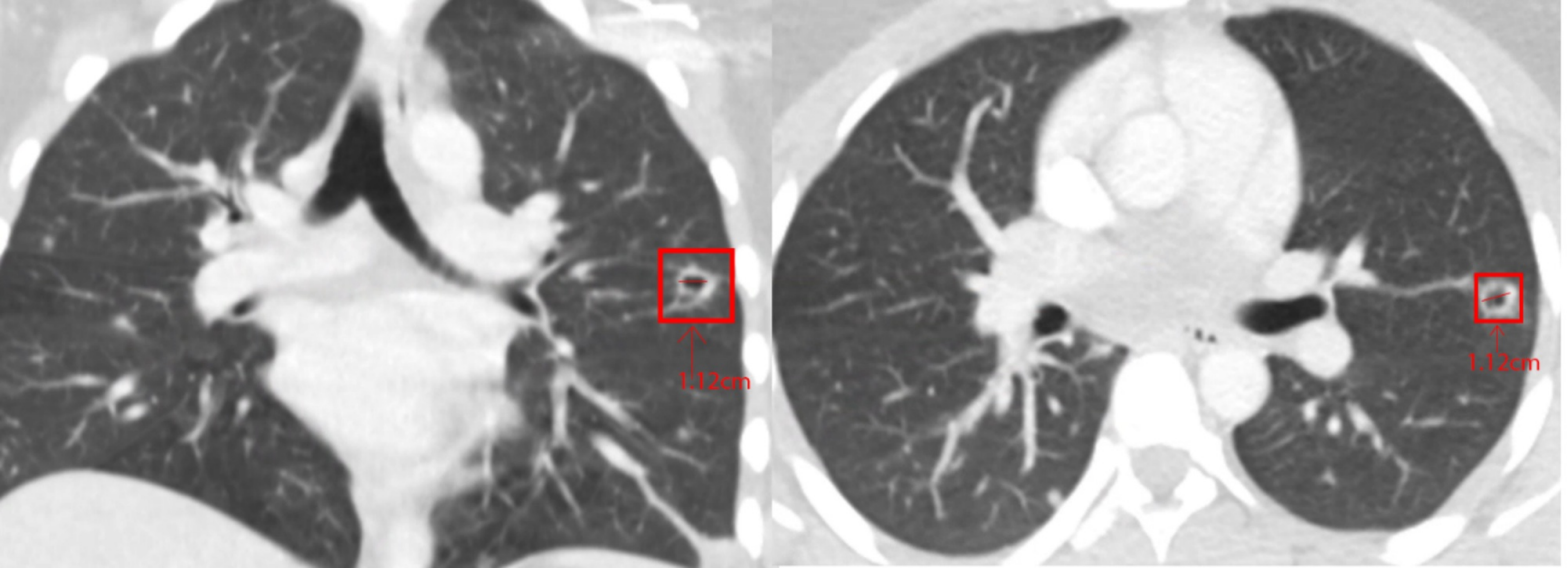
CT chest with contrast from outside hospital prior to admission showing multiple, bilateral cavitary nodules Left: coronal plane CT chest with contrast. Right: axial plane CT chest with contrast.
